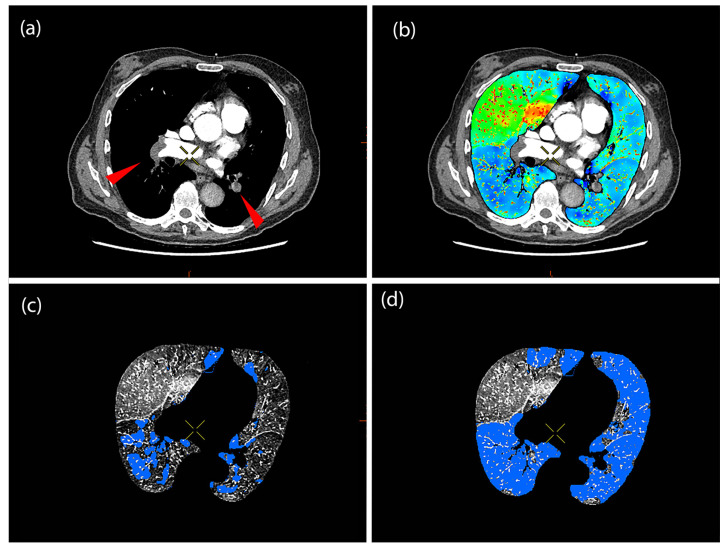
Representative example of perfusion defect assessment in a patient with bilateral basal pulmonary embolism. (a) Axial CTA image showing emboli in bilateral lower lobe pulmonary arteries (red arrowheads). (b) Corresponding iodine perfusion map from DECT, demonstrating reduced perfusion in both lower lobes and partially in the upper lobes. (c) Automated segmentation using Thoracic VCAR software, with perfusion defects shown in blue; note under-segmentation relative to the extent of visible perfusion abnormalities. (d) Semi-automated segmentation after manual threshold adjustment, showing more extensive perfusion defects involving bilateral lower lobes and anterior regions of the upper lobes, aligning more closely with the embolic distribution seen on CTA.
